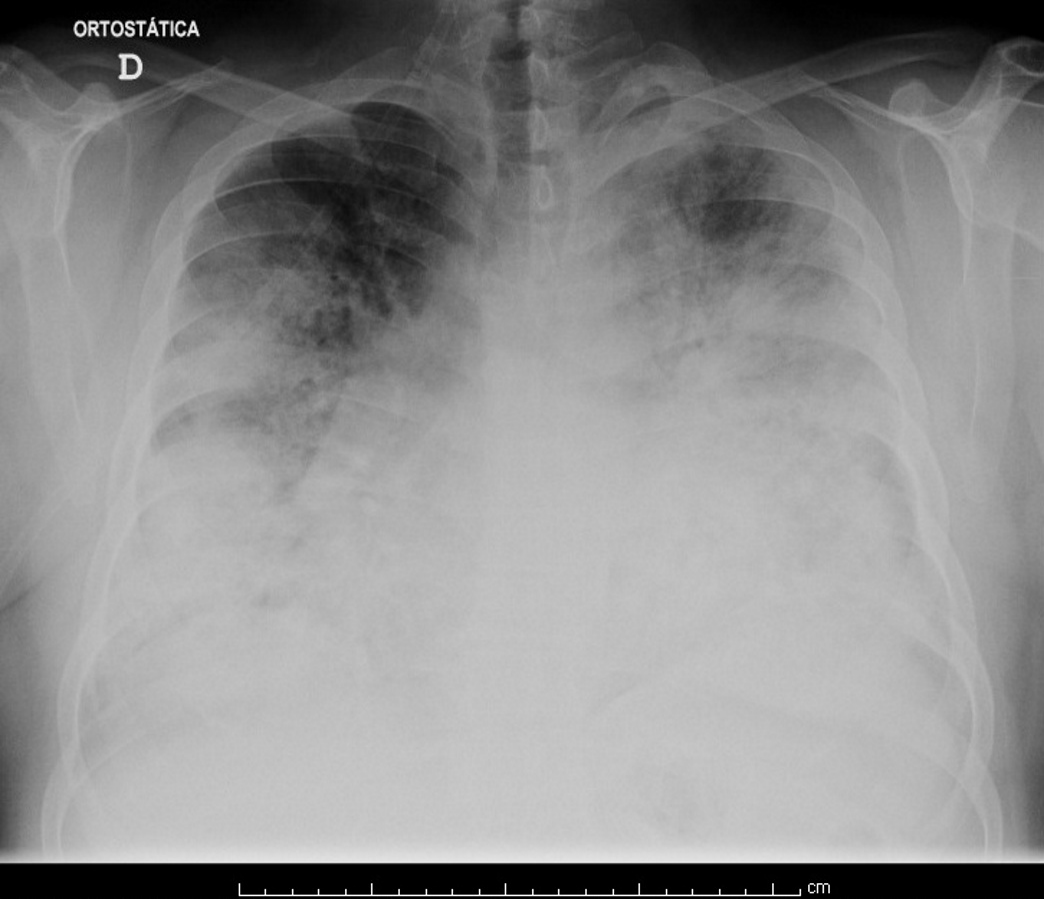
Chest radiography on admission to the Emergency Department. Extensive bilateral infiltrates involving the lower two-thirds of both lung fields can be observed.
